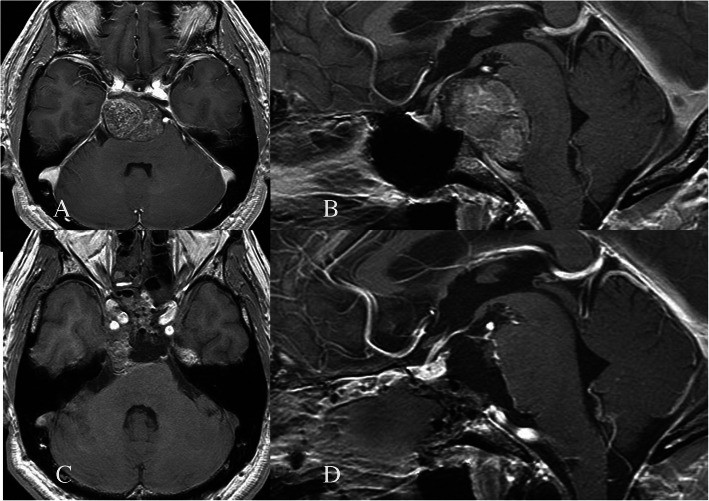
Head MR imaging with contrast medium showing massive clival tumor compressing the entire pons backward (a: axial image, b: sagittal image); surgery removed 90% of the tumor was removed except for the right retrocavernous sinus portion (c: axial image d: sagittal image)
